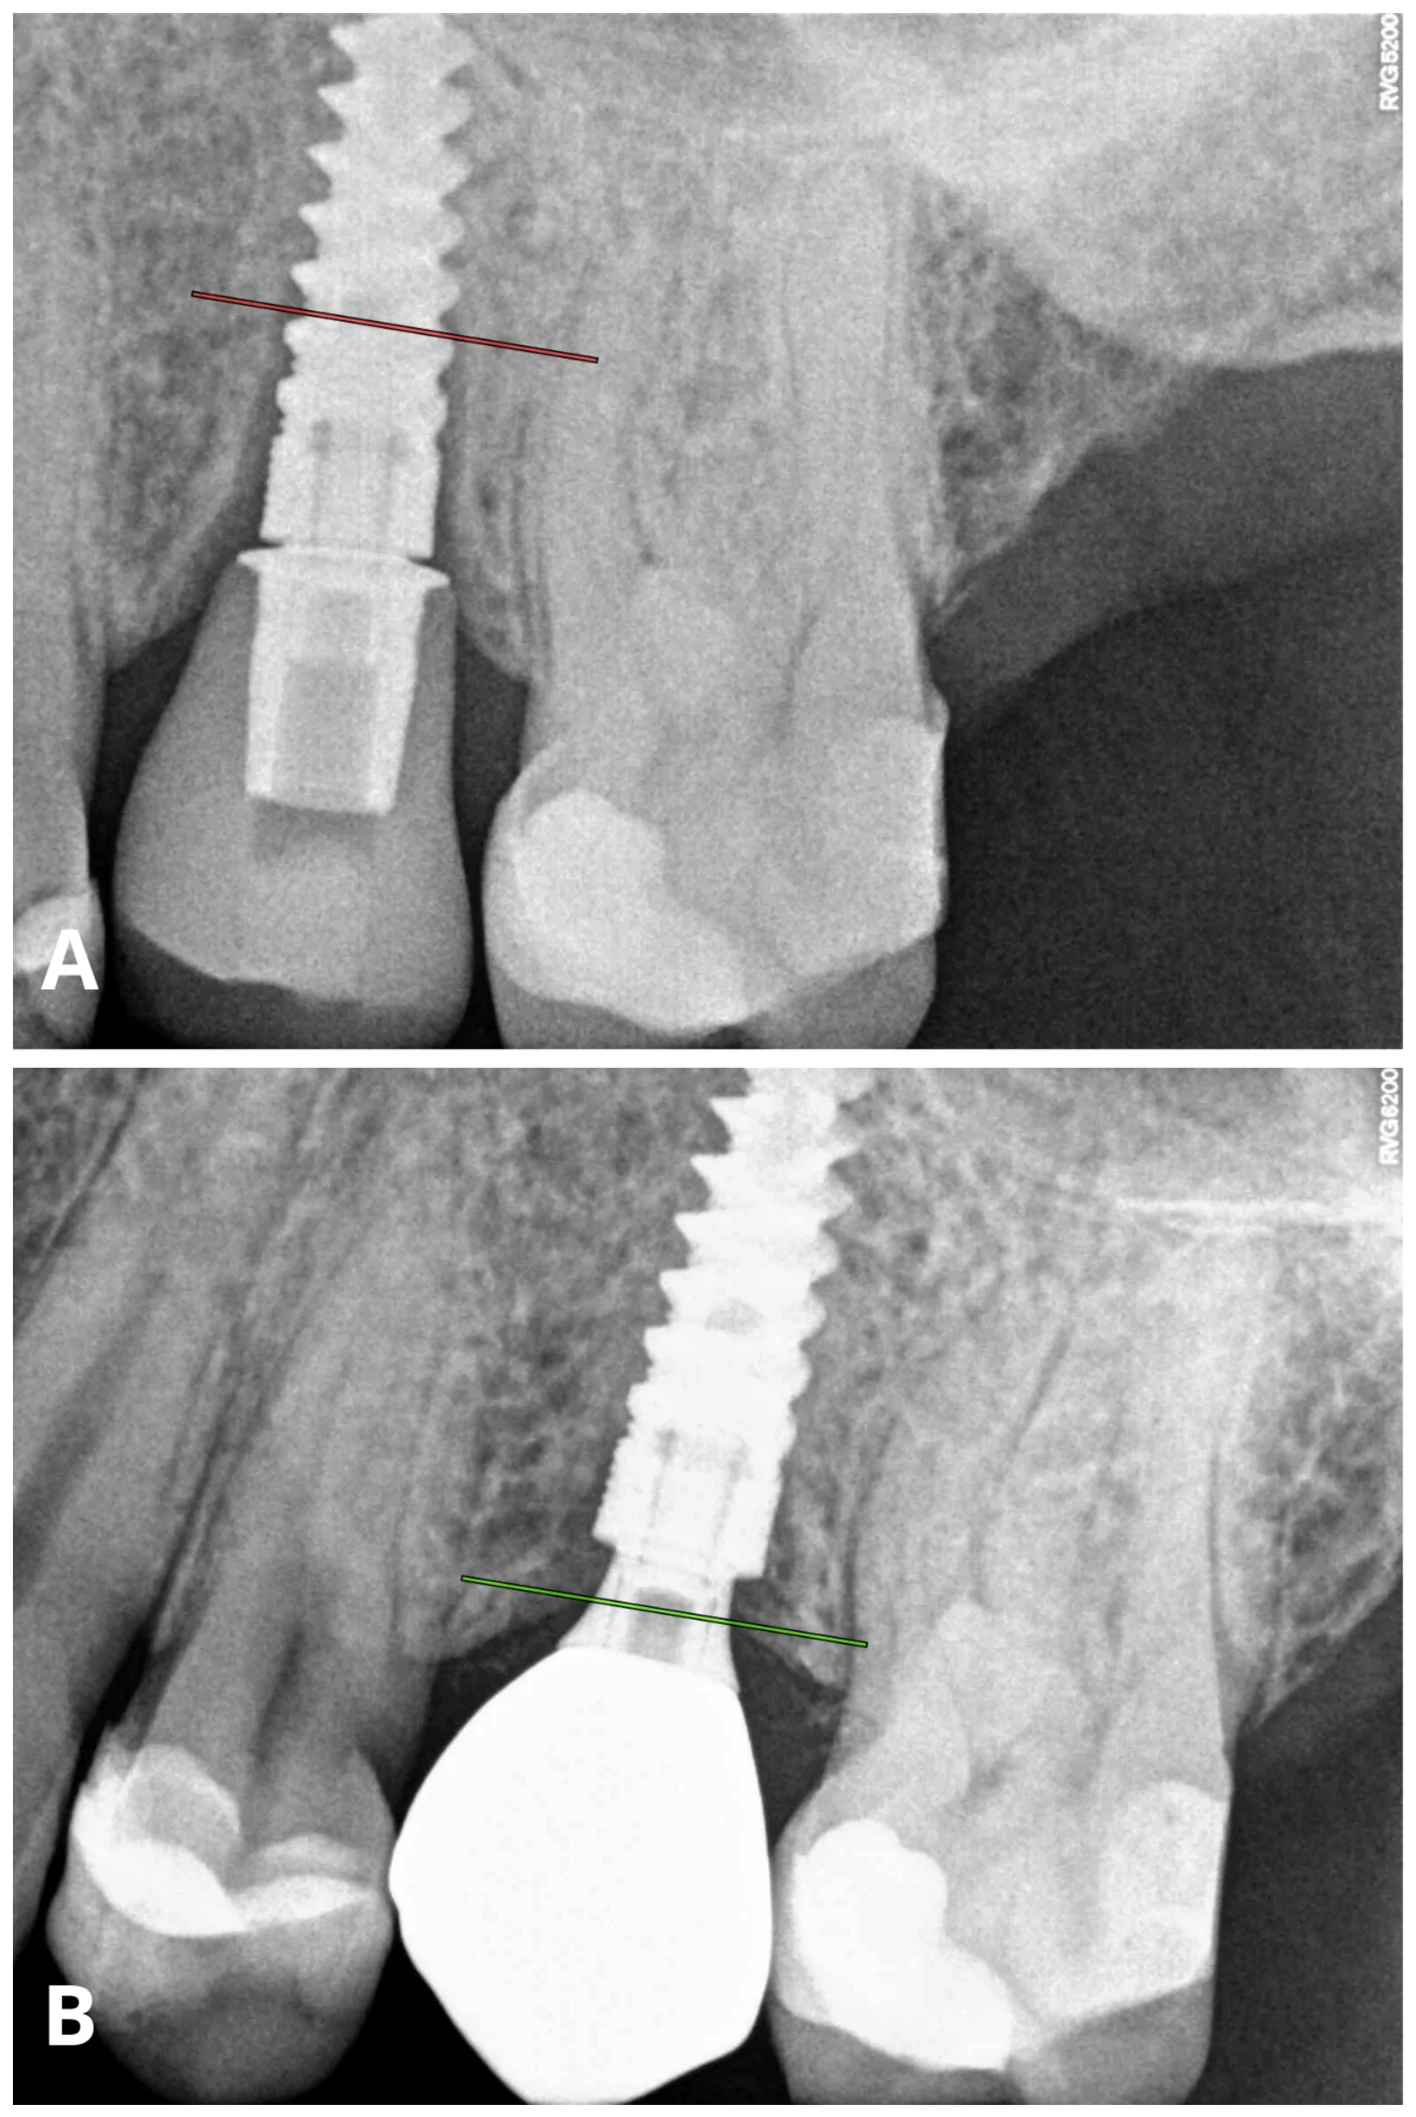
(A)—preoperative radiograph of the implant at site 25 with the level of the bone defect indicated (red line); (B)—5-year follow-up radiograph with the bone level indicated (green line).
